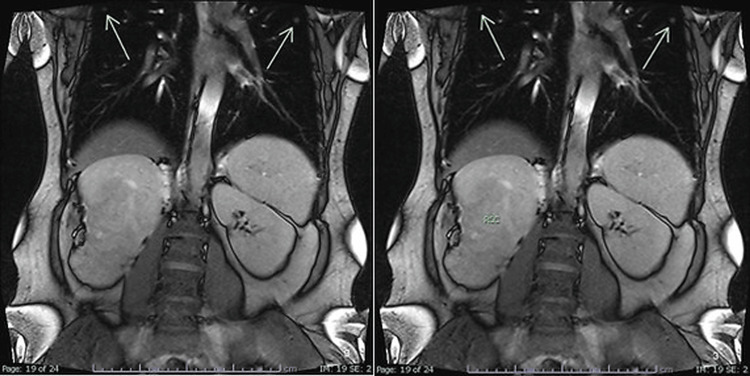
Coronal MRI images demonstrating bilateral pulmonary metastases (arrows).
